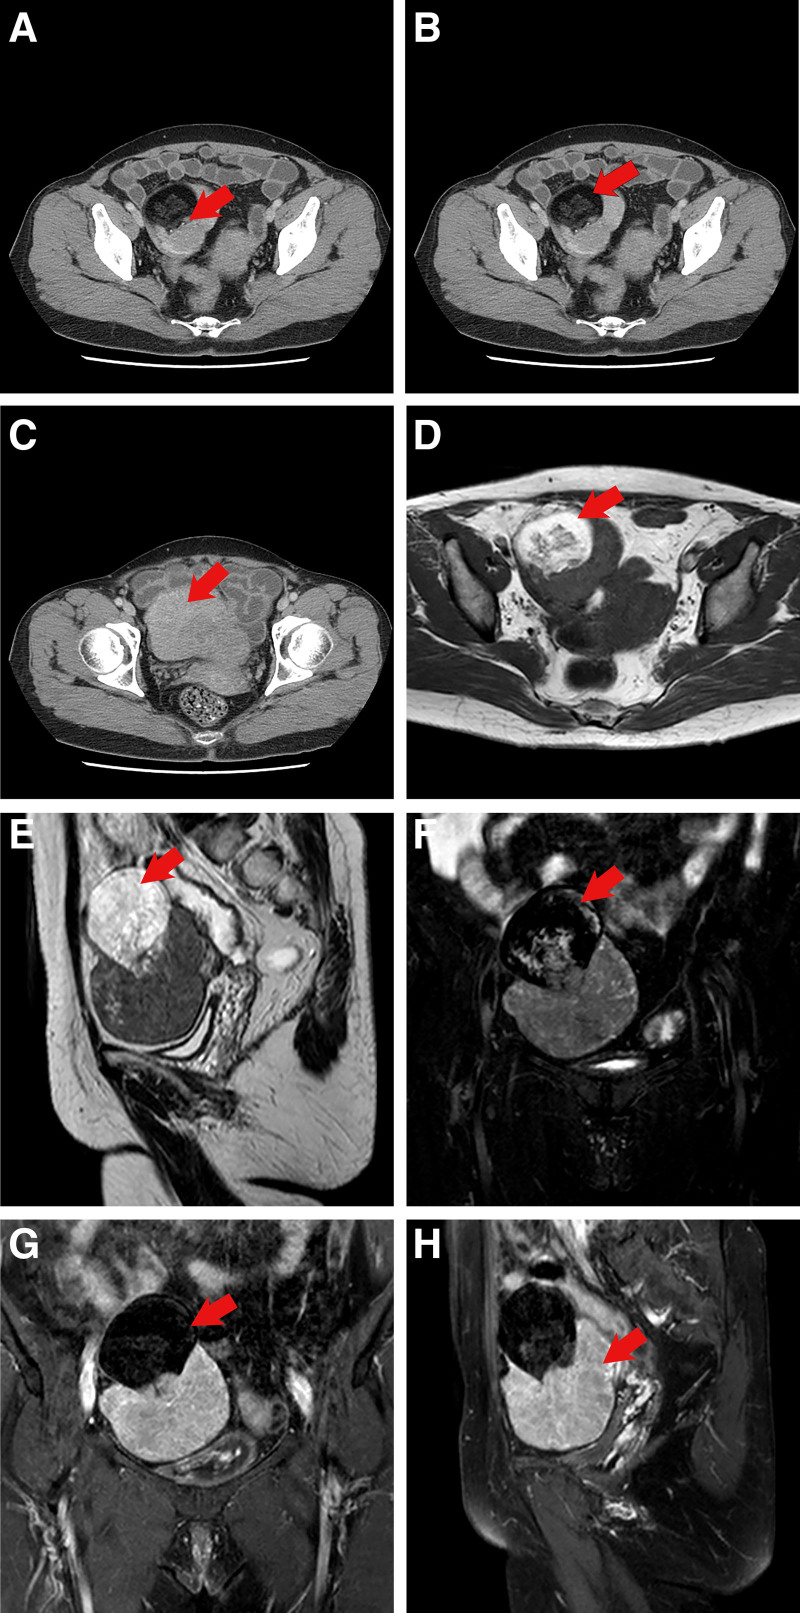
(A–H) CT and MRI imaging of the tumor. (A) A cystic-solid mixed-density shadow was seen on the right side of the pelvis, with a small amount of calcification (arrow). (B) The cystic component exhibited fat density (arrow). (C) The solid part of the lesion demonstrated marked and more homogeneous enhancement on scanning (arrow). (D) The cystic part of the T1WI sequence showed a high-signal area (arrow). (E) The cystic part of the T2WI showed a high-signal shadow (arrow). (F) The signal of the cystic part was significantly attenuated on compressed fat scanning (arrow). (G) Significant homogeneous enhancement of the solid portion following enhancement (arrow), while the cystic portion was not enhanced. (H) Significant homogeneous enhancement of the solid portion following enhancement (arrow), while the cystic portion was not enhanced.
